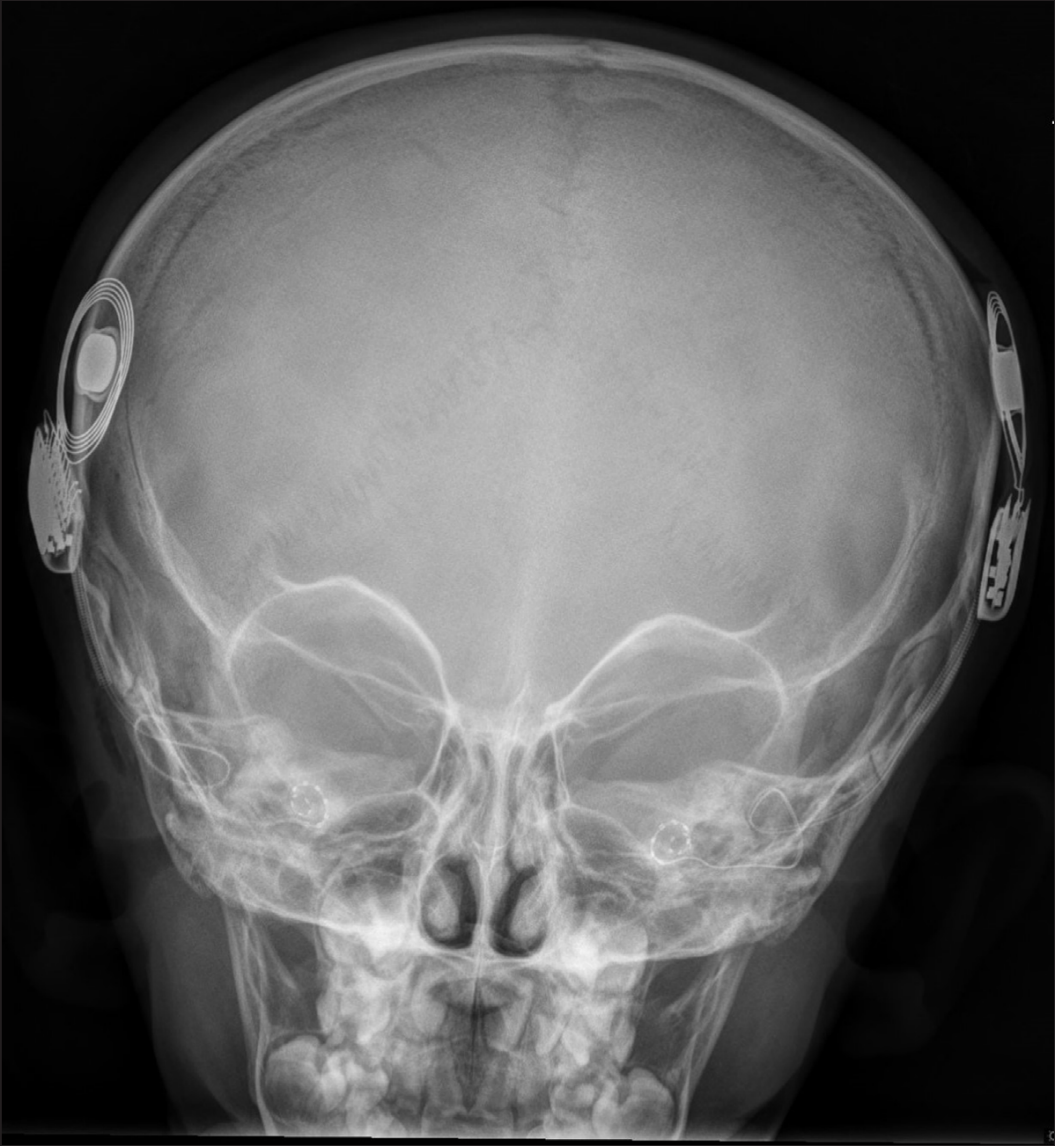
AP skull radiograph of correctly placed bilateral cochlear implants.
